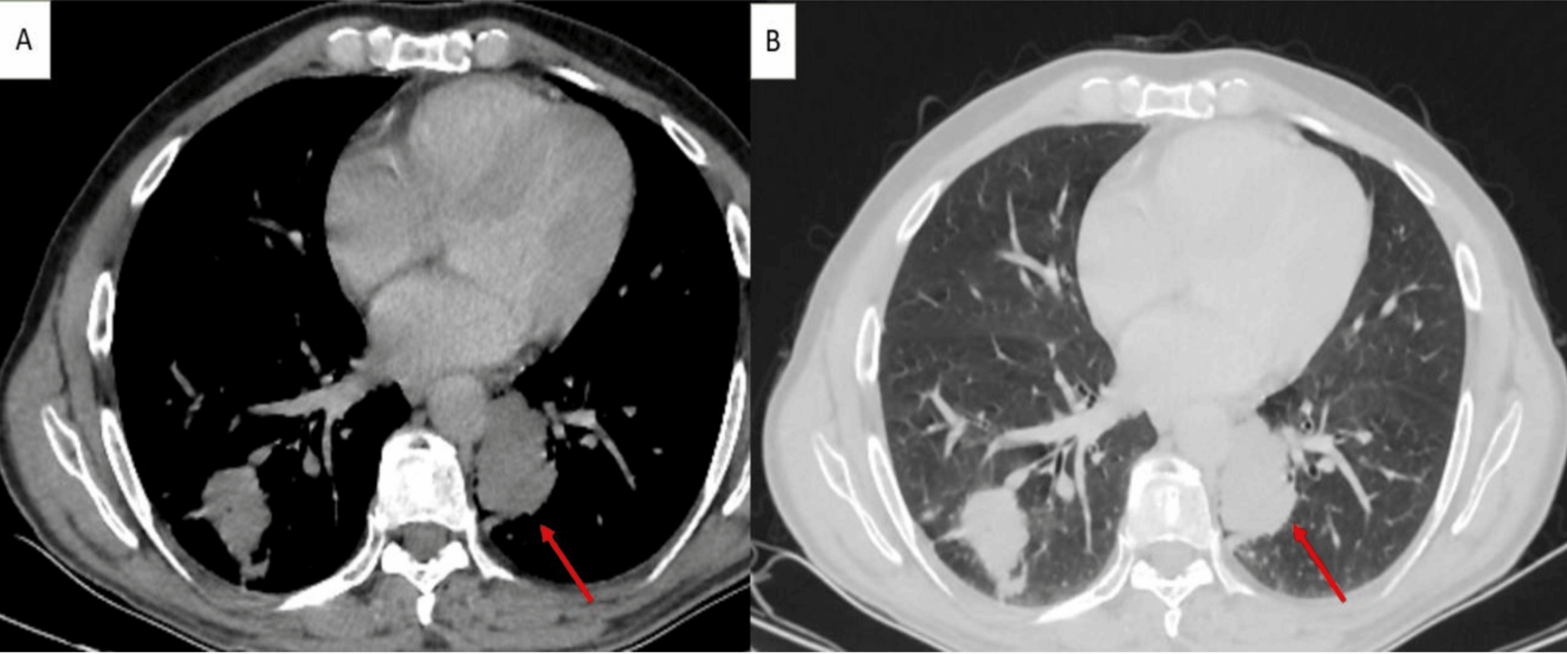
Thoracic CT scan In axial sections, displayed in mediastinal window (A) and lung window (B), after contrast injection, showing multiple bilateral and diffuse pulmonary parenchymal lesions. The largest lesion is located in the left costovertebral gutter, adjacent to the descending aorta, originating from the superior segment of the left lower lobe (red arrow), with soft tissue density and no significant enhancement after contrast administration.
